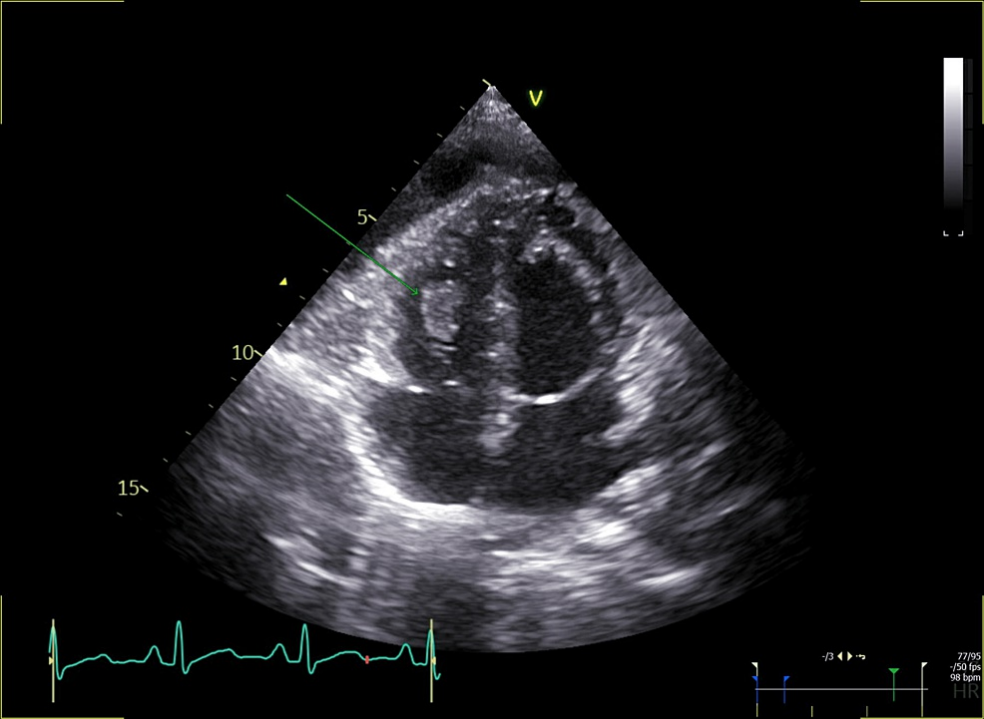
Case 2: Apical four-chamber view showing a thrombus in the right ventricle (green arrow).
